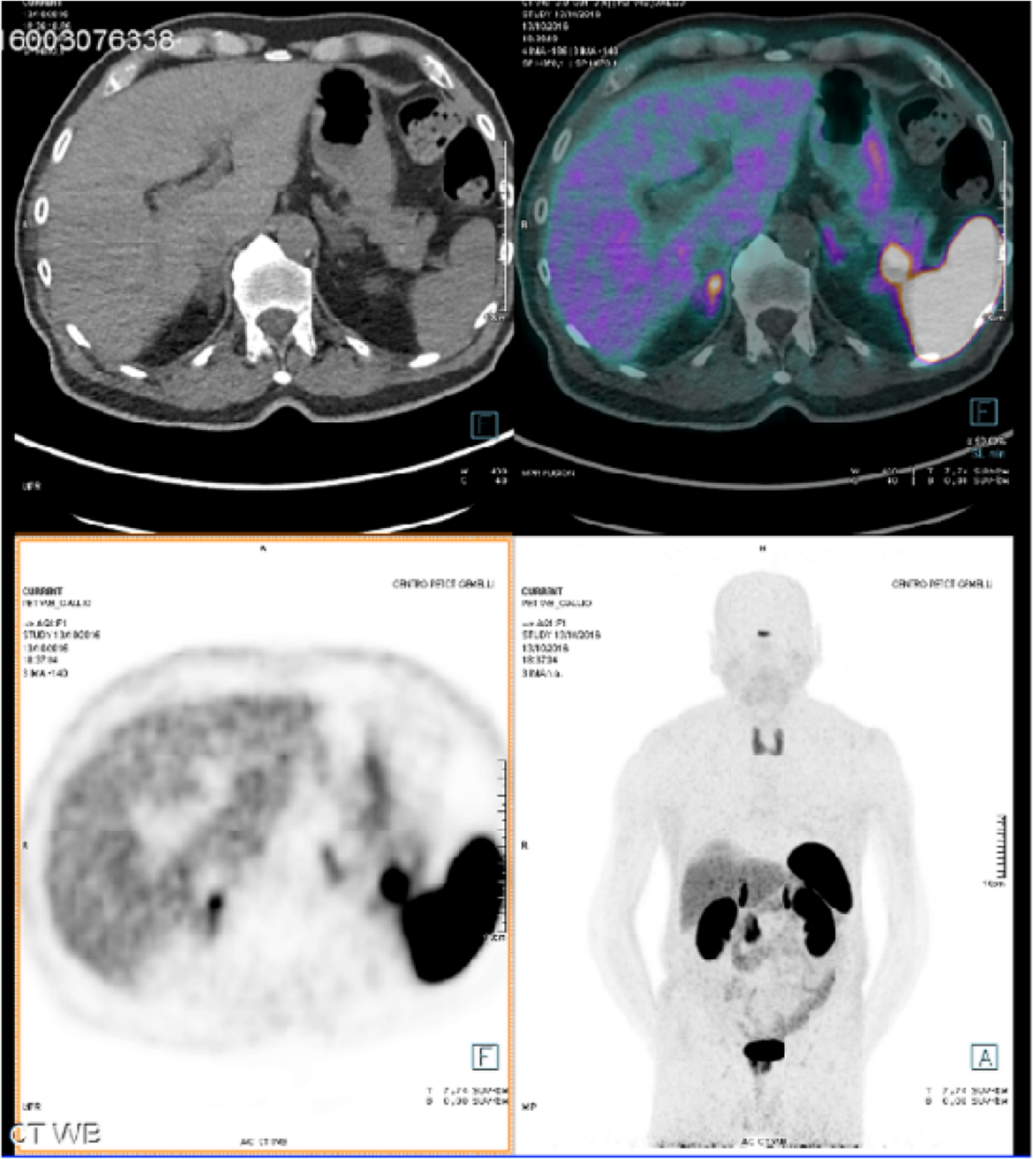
The PET image shows an indeterminate pancreatic tail nodule with enhanced uptake of 68-Ga-dotatate at PET-CT fusion image (false positive)
